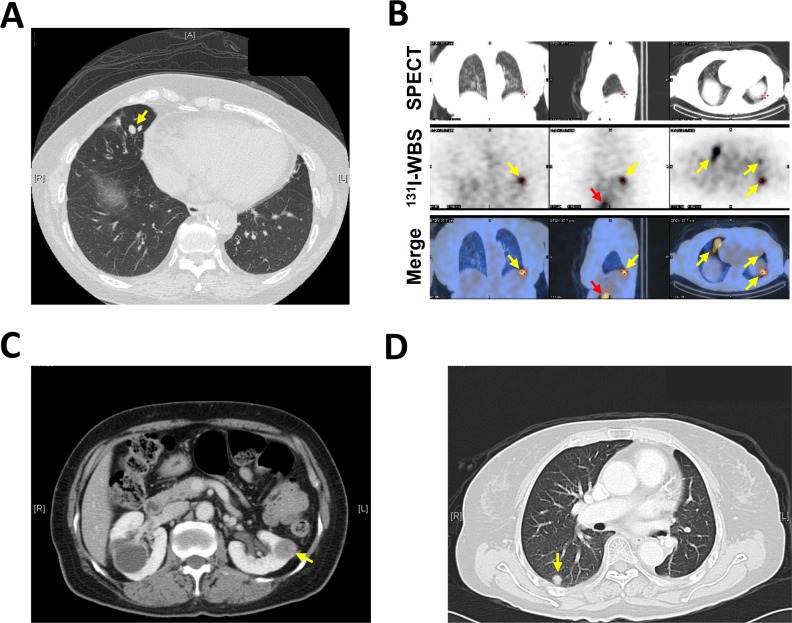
Key medical images reveal disease recurrence in cases #10 and #11 A. The image of chest CT demonstrated multiple, round but small (all less than 1 cm in diameter) pulmonary nodules (the yellow arrow indicates one of them) of case #10. These nodules were not distinguishable from the nodules derived from benign diseases such as tuberculosis. B. Single-photon emission computed tomography (SPECT, upper row) and ^131^I-WBS (middle low) were performed for case #10 at the 7^th^ day after an oral dose of 100 mCi ^131^I. The images were superimposed (bottom row) to show the multiple focal areas of increased uptake in lung (yellow arrows) and right pelvis (red arrows). The coronal view (left panel), sagittal view (middle panel) and transverse view (right panel) of the images are shown. C. The abdominal CT image for case #11 shows a left renal middle pole tumor (2.2 × 1.7 cm) which was undistinguishable from a primary renal cell carcinoma. D. The chest CT image for case #11 shows one of the multiple small and round opacities in the lung (yellow arrow).
